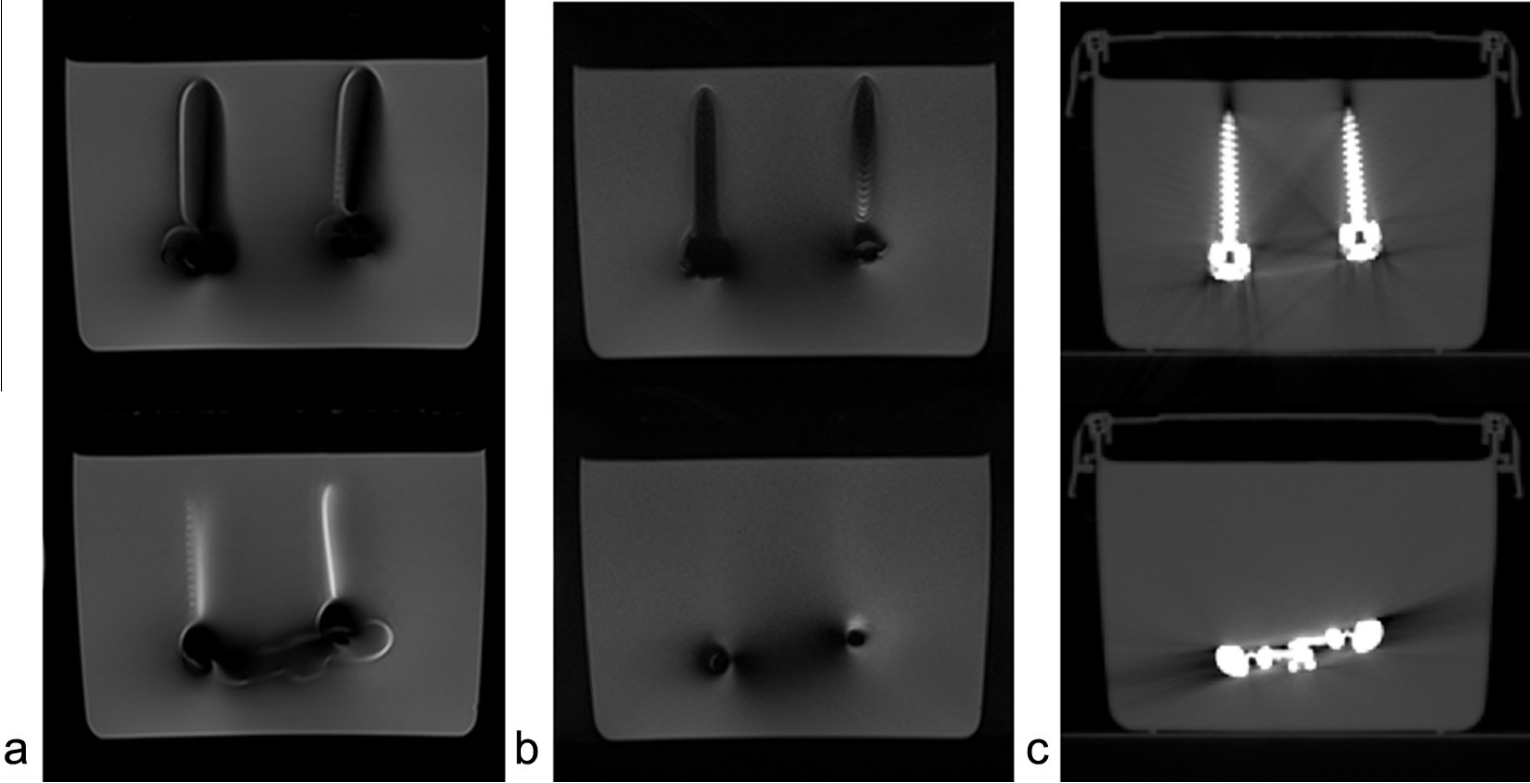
Conventional FSE (a) and SEMAC FSE (b) images for two separate slices (top and bottom). Signal loss and signal pile up are both greatly reduced with the introduction of SEMAC, with some residual effects in the vicinity of the implant. CT images (2.5 mm slice thickness) at approximately the same location are shown for comparison (c).
